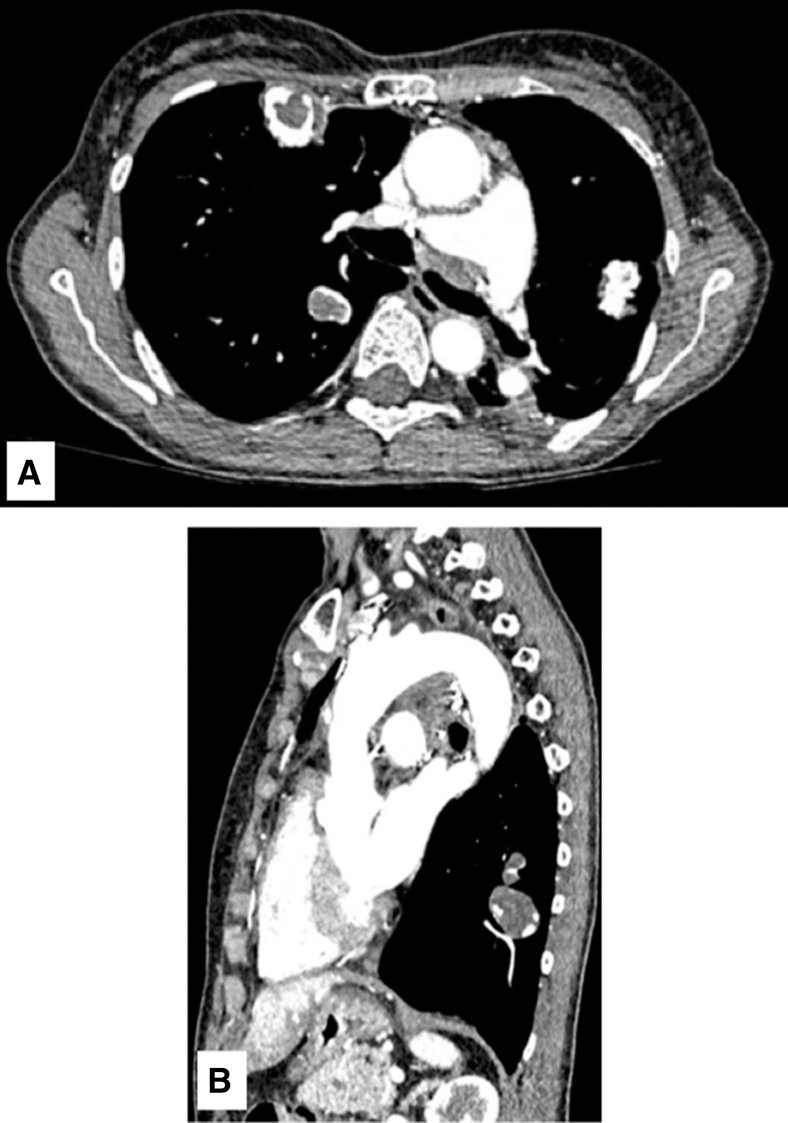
Contrast-enhanced computed tomography scan of the chest at 10 months postoperatively in axial (A) and sagittal (B) views showing the tumorectomy site with restoration of the normal anatomy of the aortopulmonary window.
